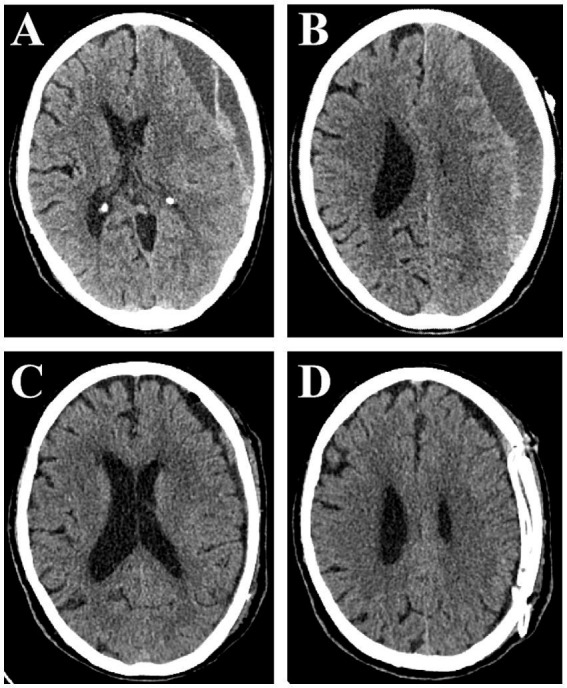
Computed tomography scan showing septated chronic subdural hematoma on the left side. Small red arrows indicate SCSH with two clear compartments (A,B). Early postoperative scan showing significant clearance of hematoma, regression of midline shift, and slight intracranial pneumatosis (C,D).
